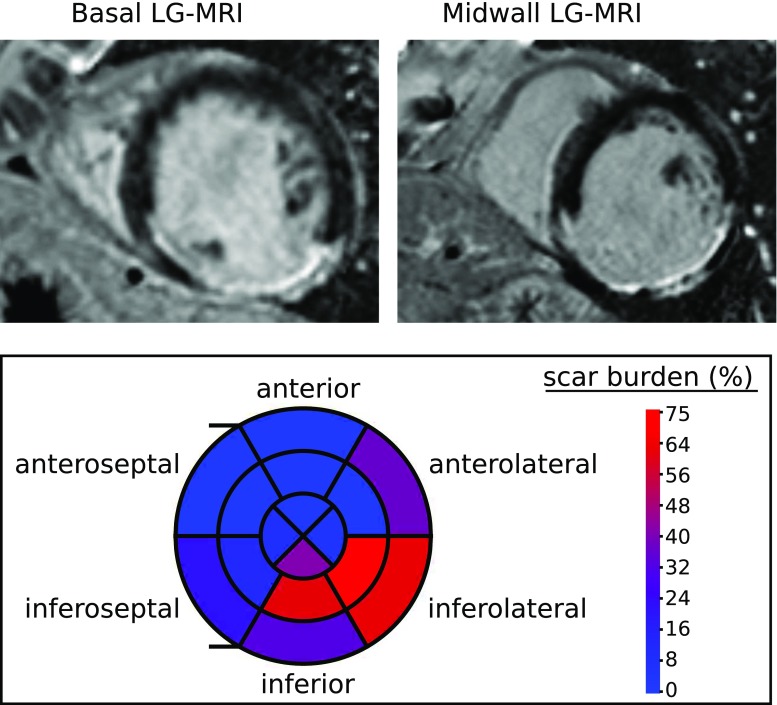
Top row: two example short-axis late enhancement gadolinium MRI images used for regional scar quantification. Fibrotic sections of the myocardium appear in white. Bottom row: regional quantification of myocardial scar burden based on LG-MRI. The inner, middle, and outer rings represent apical, midwall, and basal sections, respectively. The RV insertion points are marked by two horizontal lines extending to the left of the bullseye
